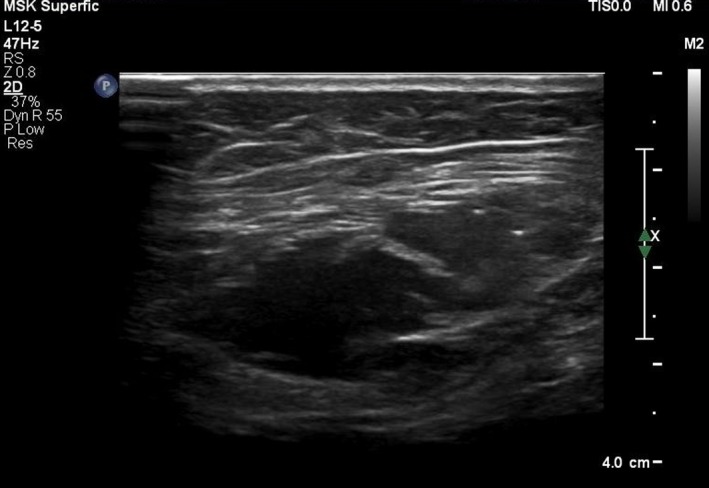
Ultrasound guided injection of 300 U of Botox along the superficial fascia of the transversus abdominis, internal and external oblique muscles bilaterally at three levels; above the iliac crest, at the level of the umbilicus, and below the costal margin
